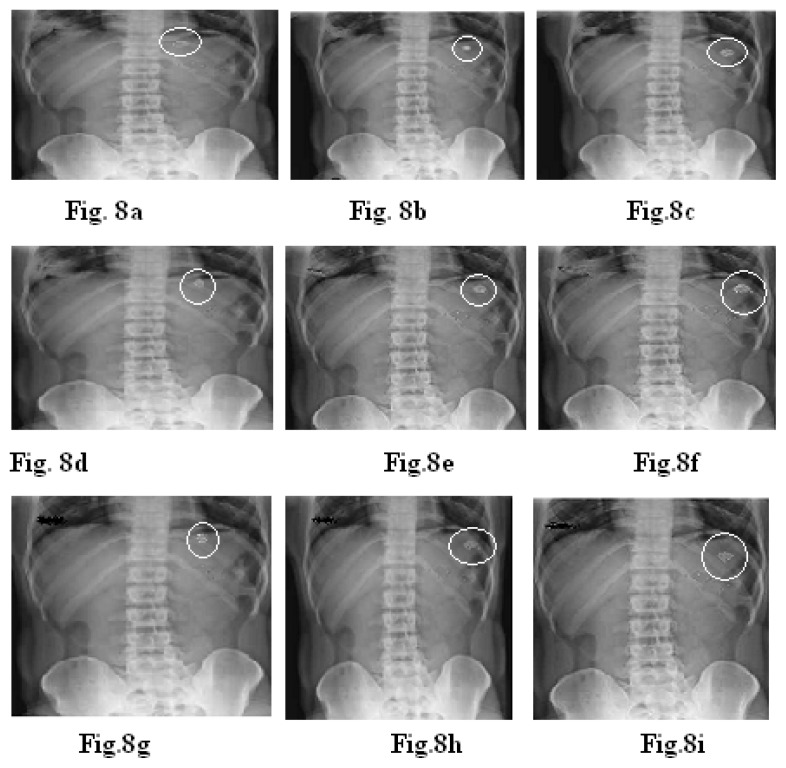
X-rays indicating the position of the floating tablet in the gastrointestinal tract of volunteers for the formulations C2+B4, C6+B4, and C9+B4. X-ray taken at 15 min (a,d,g), at 2 hrs (b,e,h), and at 4 hrs (c,f,i).
